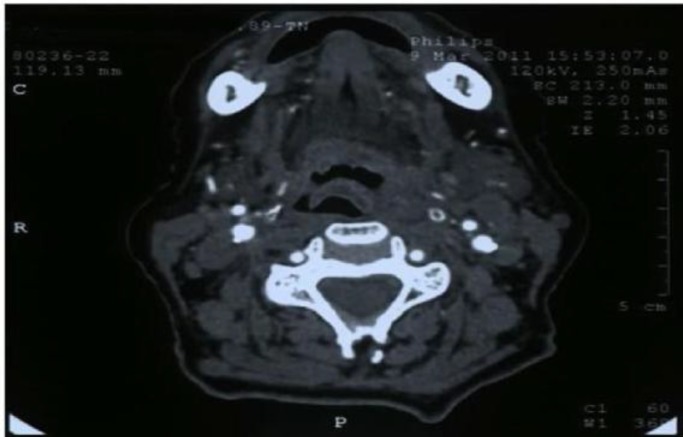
Axial computed tomography (CT) scan of neck showing a left pyriform sinus mass
